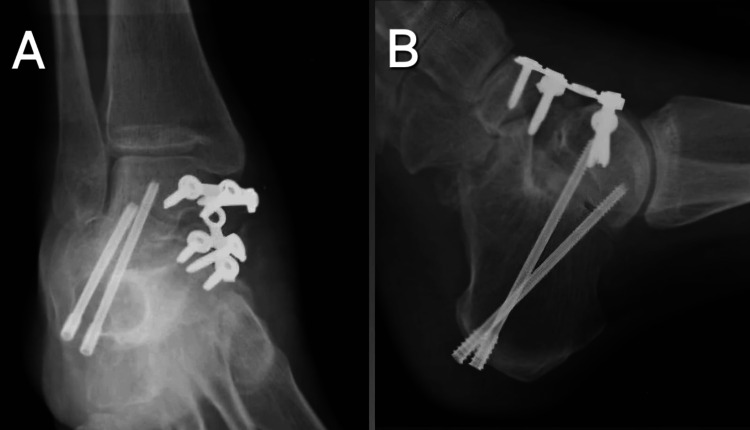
Post-operative radiograph of the right ankle in (A) anteroposterior and (B) lateral views
